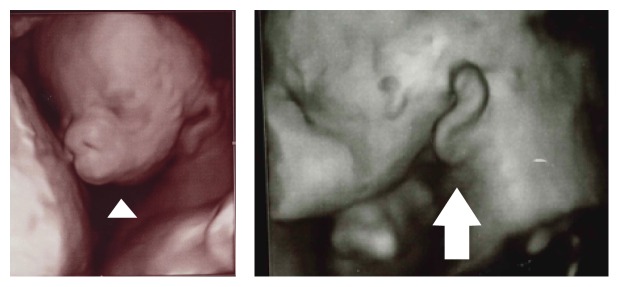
Three-dimensional ultrasonography demonstrated ventromedial displacement of the ear (arrow), small opening of the mouth, and mandibular agenesis (arrowhead).
